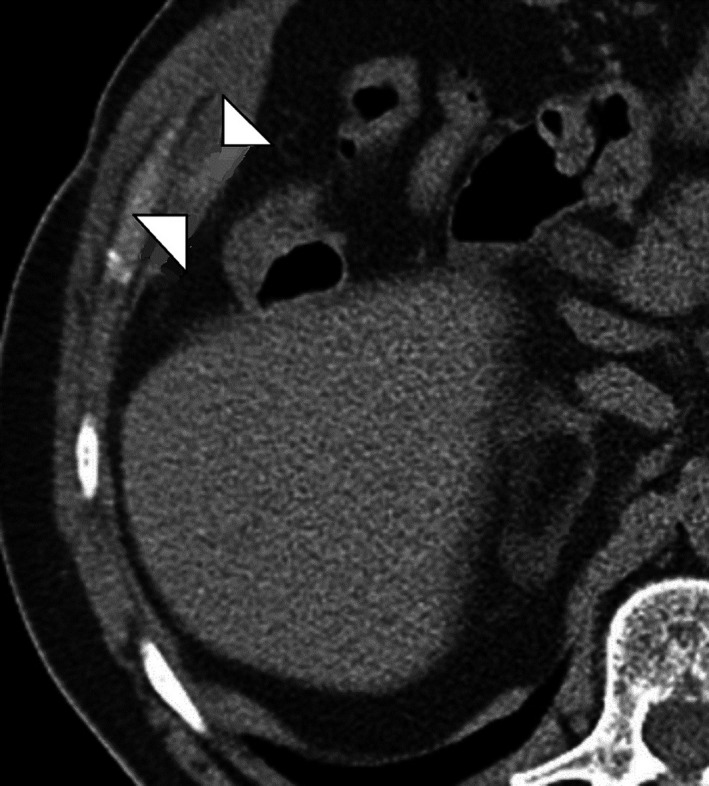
Axial section of contrast enhanced CT scan, showing evidence of small walled off collection within the subhepatic peritoneal cavity, marked between the two arrow heads.
